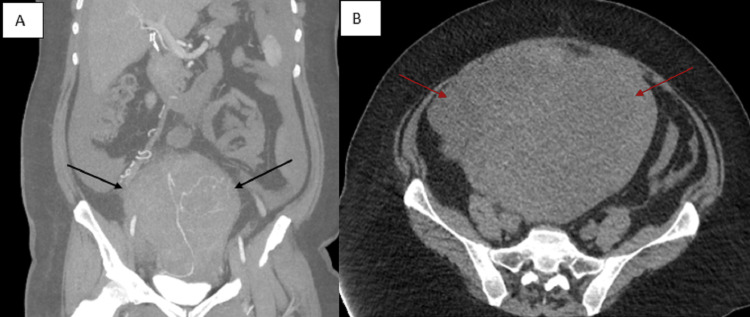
Contrast-enhanced computed tomography of the abdomen and pelvis. (A) Sagittal view with enlarged uterus (black arrows); (B) coronal view with enlarged nodular uterus (red arrows)
